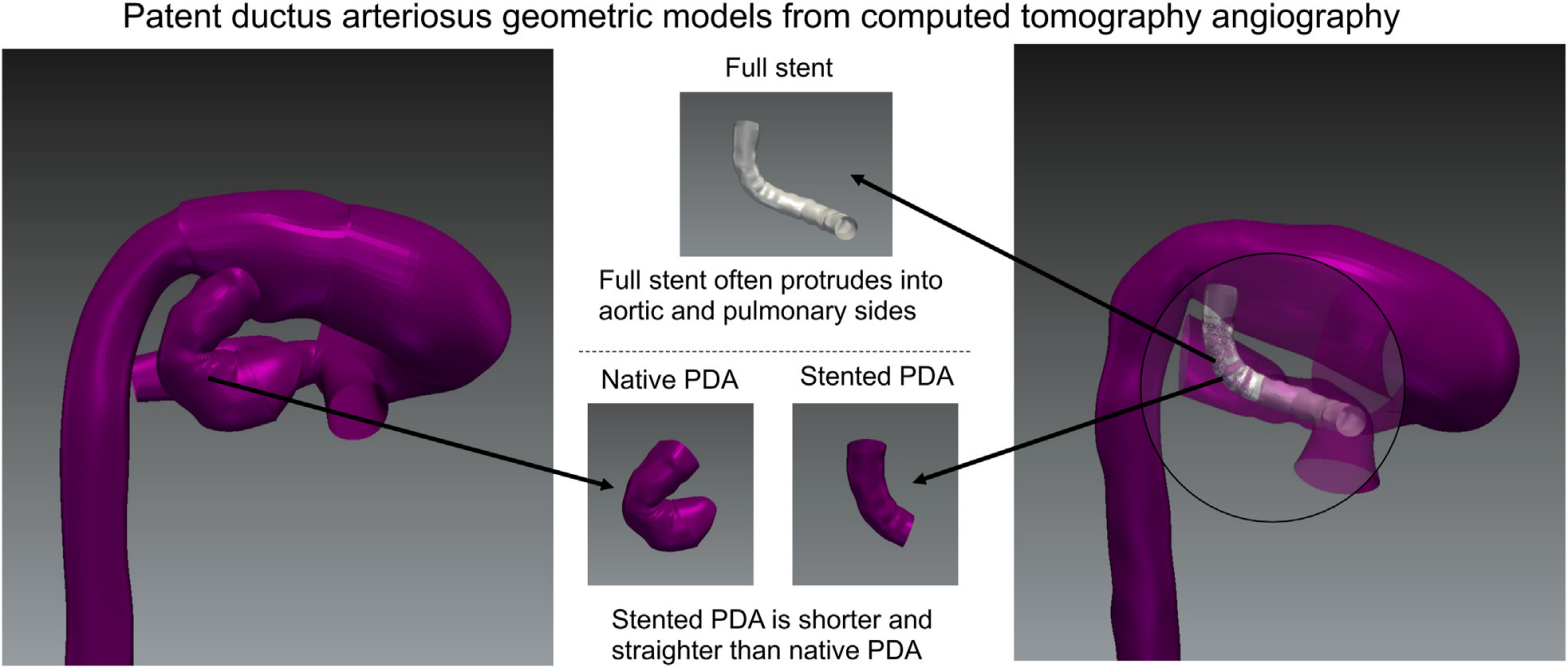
Patent ductus arteriosus geometric models from computed tomography angiography. Geometric models of the native aorta, pulmonary arteries, and native- (left) and stented- (right) patent ductus arteriosus (PDA). The full stent is visualized in gray and is often protruding into the aortic and pulmonary sides (middle-top and right). From presenting to poststenting the PDA was significantly straightened and shortened, as visualized (middle-bottom).
